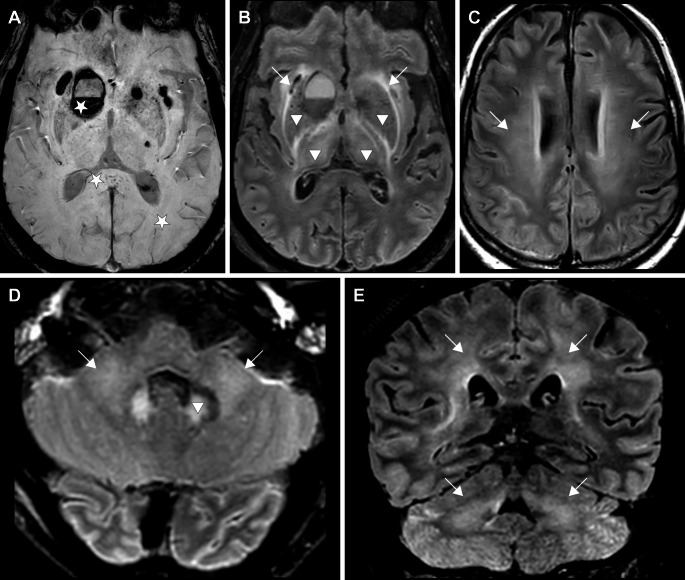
70-year-old man with a SARS-CoV–induced acute respiratory distress syndrome, requiring mechanic ventilation, experiencing difficulties weaning from sedation and confusion. Axial SWI (a), axial FLAIR (b, c, d), coronal FLAIR (e) weighted MR images. SWI hypointensities likely represent microbleeds (a, star) and intraparenchymal hematomas (a, arrowhead). Deep cerebral nuclei, thalamic and dentate nuclei FLAIR hyperintensities (b, d; arrowheads). Extensive, ill-defined, and confluent white matter FLAIR hyperintensities in subcortical, periventricular, and middle cerebellar peduncle regions (b, c, d, e; arrows)
